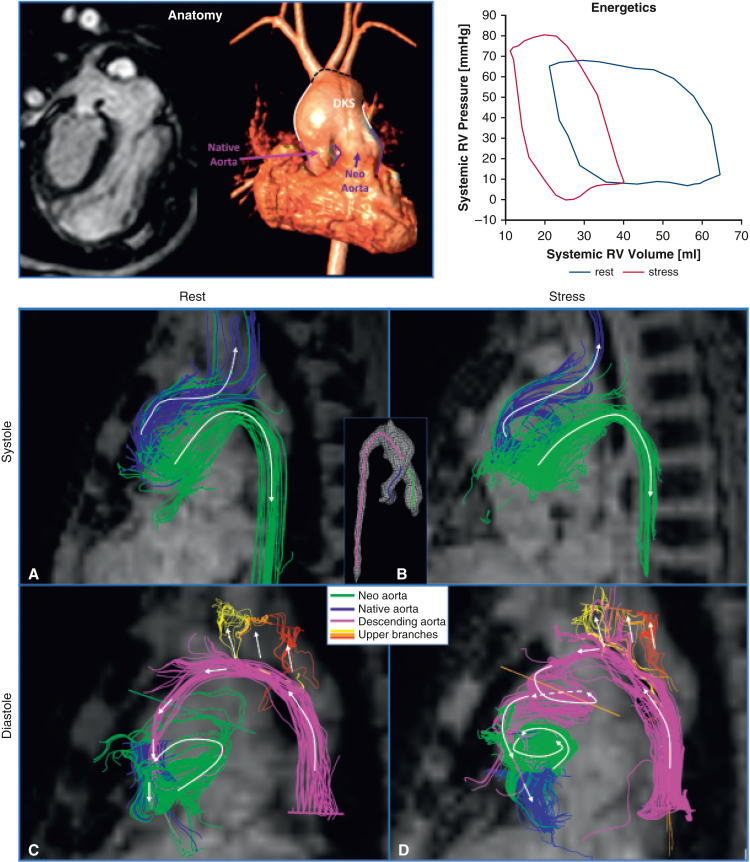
A-D, Flow distribution from 4D Flow MRI pathlines colored based on flow origin for rest (A and C) and stress (B and D) conditions during systole (A and B) and diastole (C and D). *MRI*, Magnetic resonance imaging.
